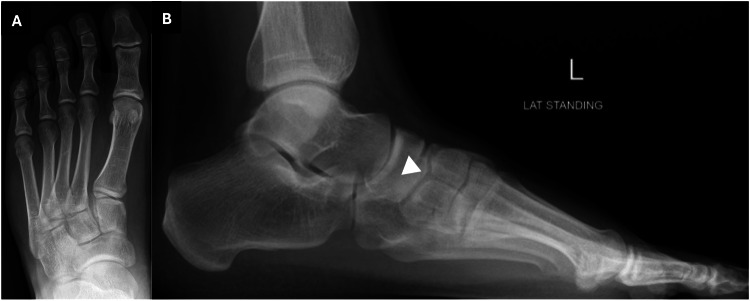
Weight-bearing radiographs of the left foot in anteroposterior view (A) and lateral view (B) showing bipartite medial cuneiform (white arrowhead).
